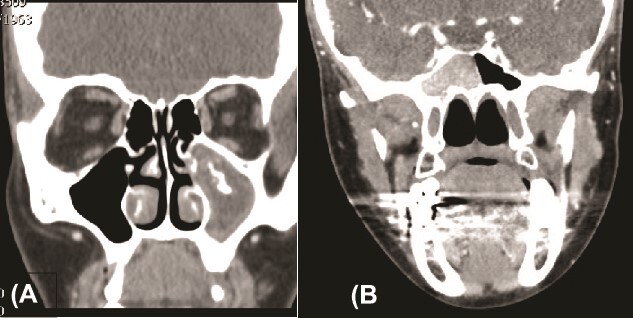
Paranasal sinuses CT scan, coronal view, showing left maxillary sinus fungal ball (A) and a right sphenoid fungal ball (B).
